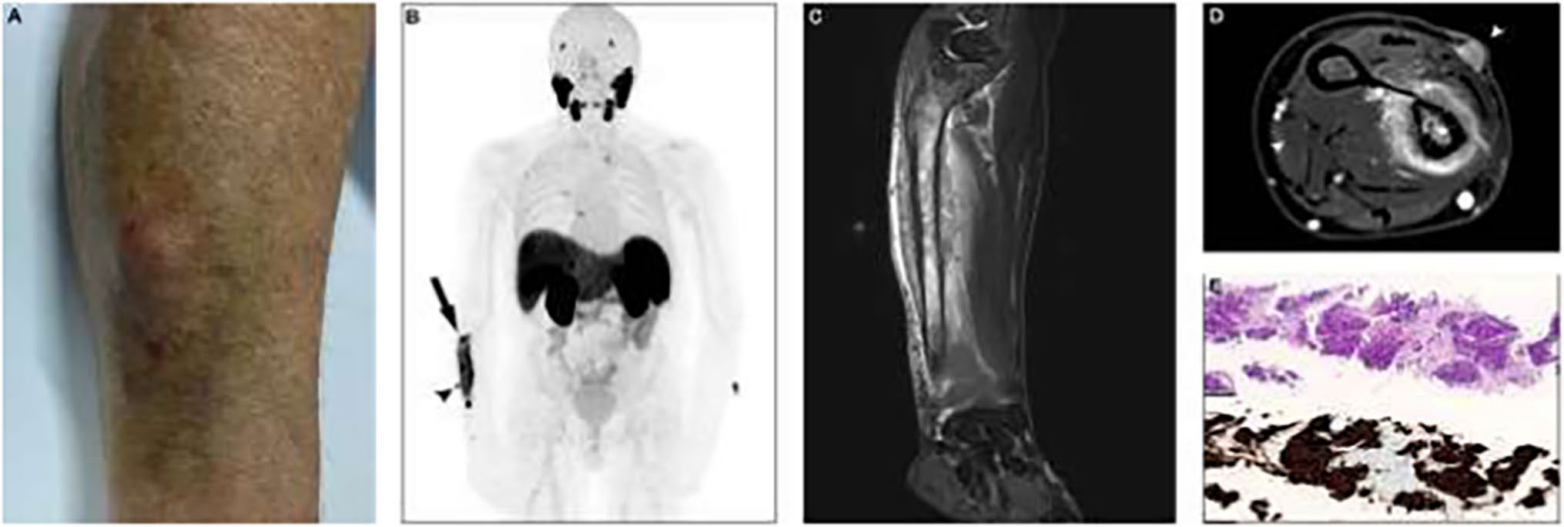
A 71-year-old man with a history of prostatectomy for prostate cancer (PCa) followed by multiple recurrences presented with a biochemical recurrence of PCa (PSA 1,3 ng/mL). Initial Gleason Score was 3 + 4 = 7a. In addition, the patient suffers from chronic lymphocytic leukemia. [^18^F]F-PSMA- 1007PET/CT was performed in accordance with standard procedure and yielded no abnormality [1]. During clinical follow-up two months later, the PSA level increased (7,9 ng/mL) and the patient complained of bruise on the left forearm.
